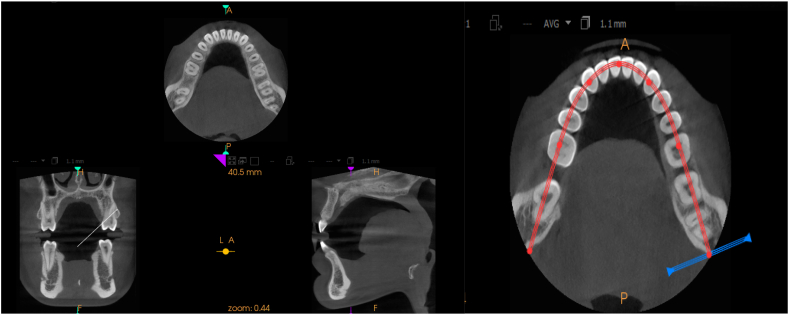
CT image for 3D model creation.
